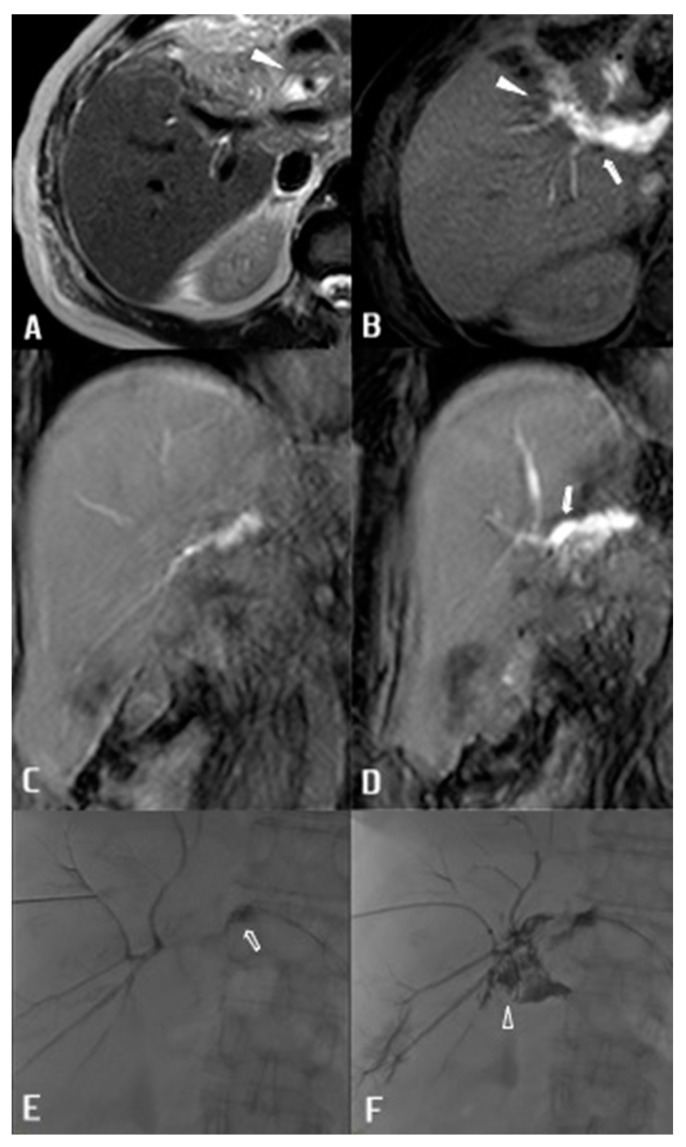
72 y.o. female patient with left hepatectomy and double bilio-enteric anastomoses. (A) T2-weighted axial image shows site two sites of the anastomoses with fluid collection medially to the intestinal loop (arrowhead). (B) T1-weighted axial image obtained 20 min after administration of c.m. demonstrates opacification of the right posterior (arrow) and right anterior (arrowhead) anastomotic ducts and opacification of the fluid collection across the surgical drainage tube. (C) T1-weighted coronal image acquired 20 min after administration of c.m. demonstrates right anterior anastomosis with opacification of bowel loop. (D) T1-weighted coronal image acquired 20 min after administration of c.m. demonstrates right posterior anastomosis with clear evidence of cm leakage (arrow). (E,F) Percutaneous cholangiography after puncture of a sub-segmentary VIII segment duct confirms leakage at the anastomotic site with precocious opacification of fluid collection across the drainage tube and delayed opacification of the intestinal loop (arrowhead).
